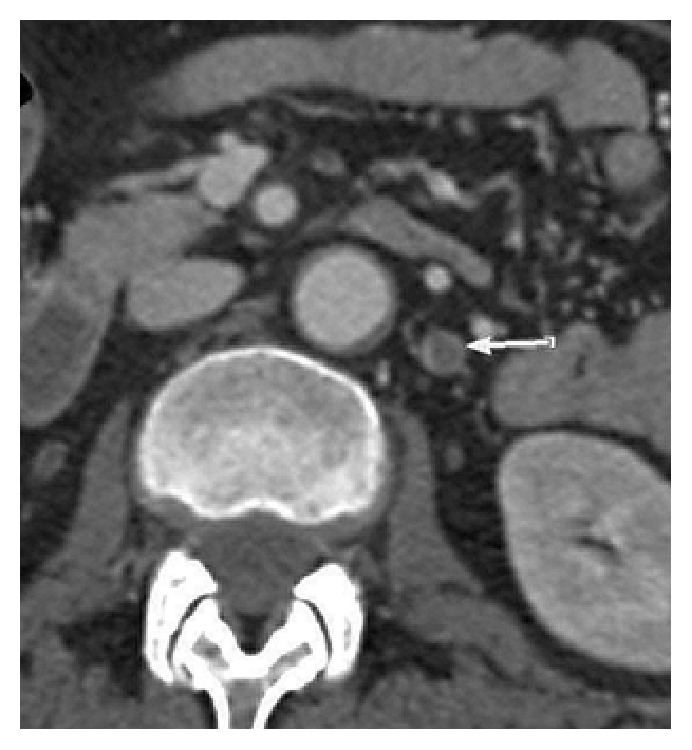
Axial view of CT showing wall enhancement of right ovarian vein in the portal venous phase, with a low density lesion representing the thrombus.
